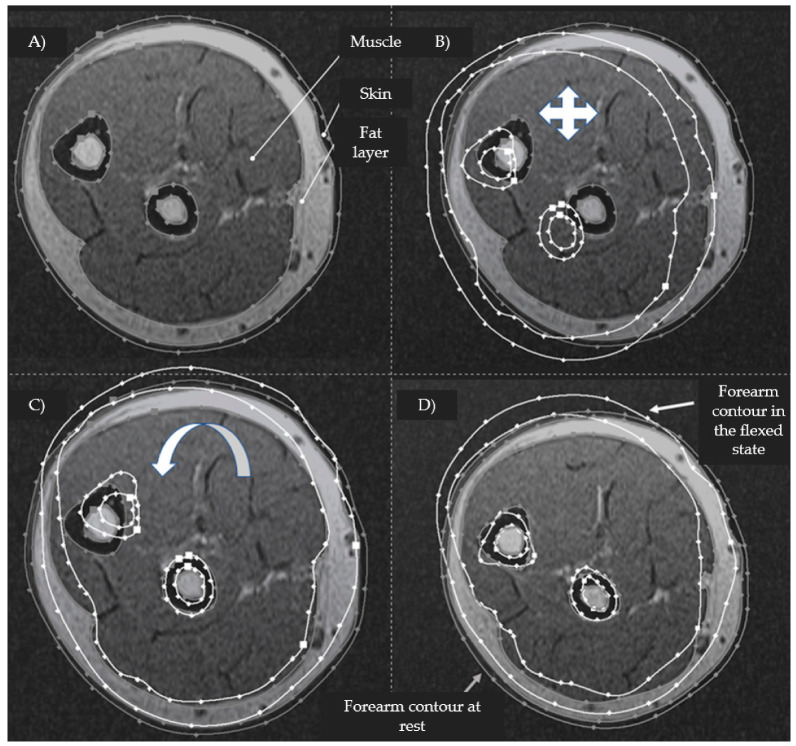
An example of contouring the skin-fat, muscle, and bone layer according to an MRI image and applying the corresponding contours (gray contour—the contour of the forearm at rest; white contour—the contour of the forearm in a state of flexion) after combining the outlines of the ulna and radius bones ((A)—contouring the layers using the “Spline” function; (B)—superimposition of the corresponding sections; (C)—alignment along the outlines of the radius; and (D)—alignment along the outlines of the ulna).
